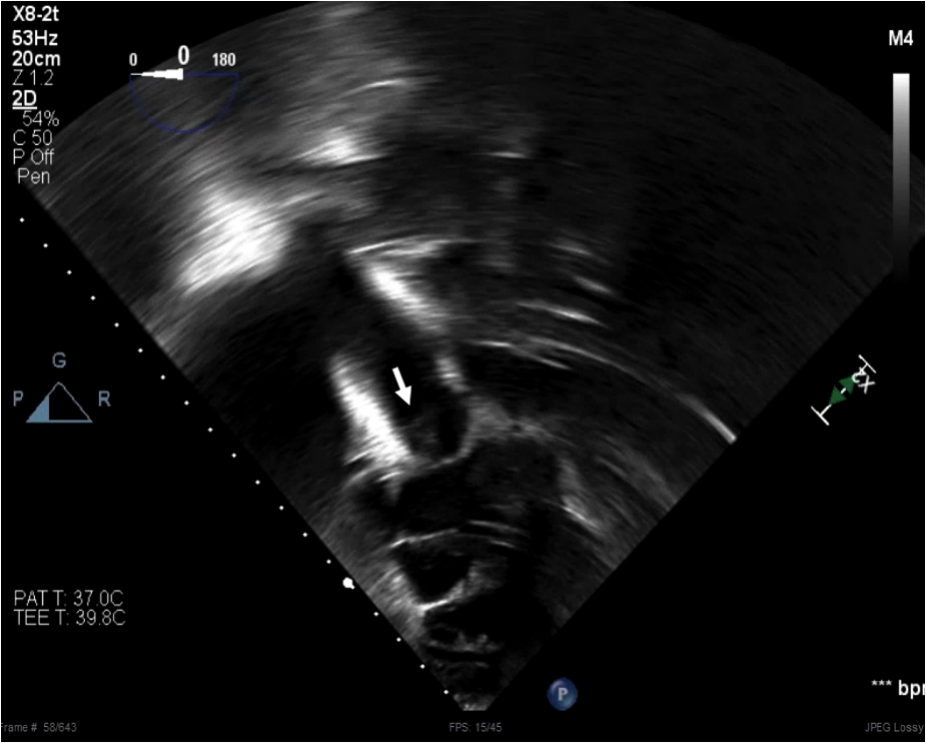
A mobile structure (arrow) visualized in the pulmonary valve from trans-gastric view on transesophageal echocardiogram. The structure moved with the valve, well attached to the leaflets.
